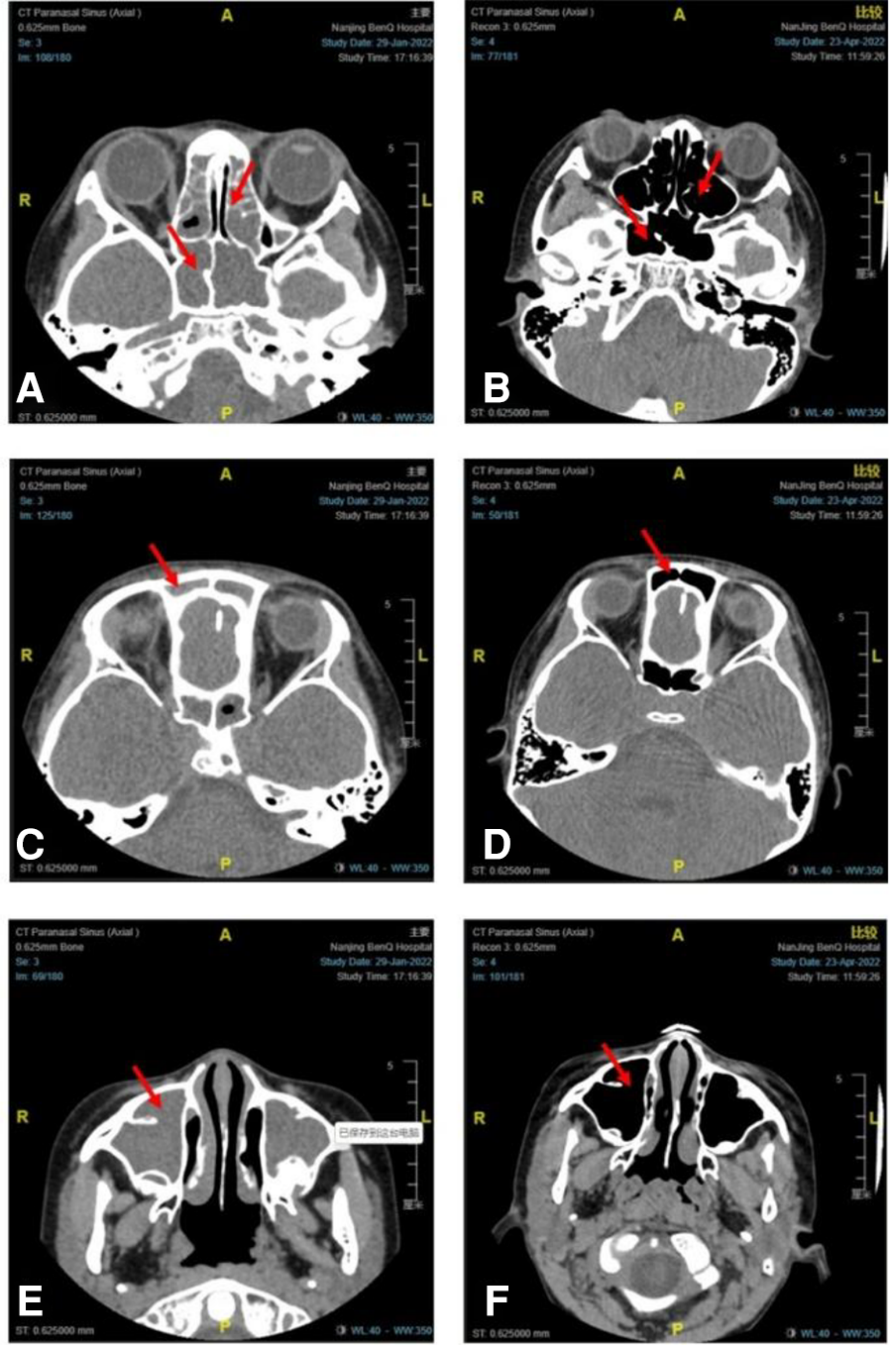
Representative CT scans of sinuses in a 9-year-old boy with moderate-to-severe allergic asthma combined with chronic sinusitis before and after 16 weeks of omalizumab treatment. CT scans of the sphenoid sinus and ethmoid sinus before (A) and after (B) omalizumab treatment. CT scans of the frontal sinus before (C) and after (D) omalizumab treatment. CT scans of the maxillary sinus before (E) and after (F) omalizumab treatment. Red arrows indicate sinusitis.
